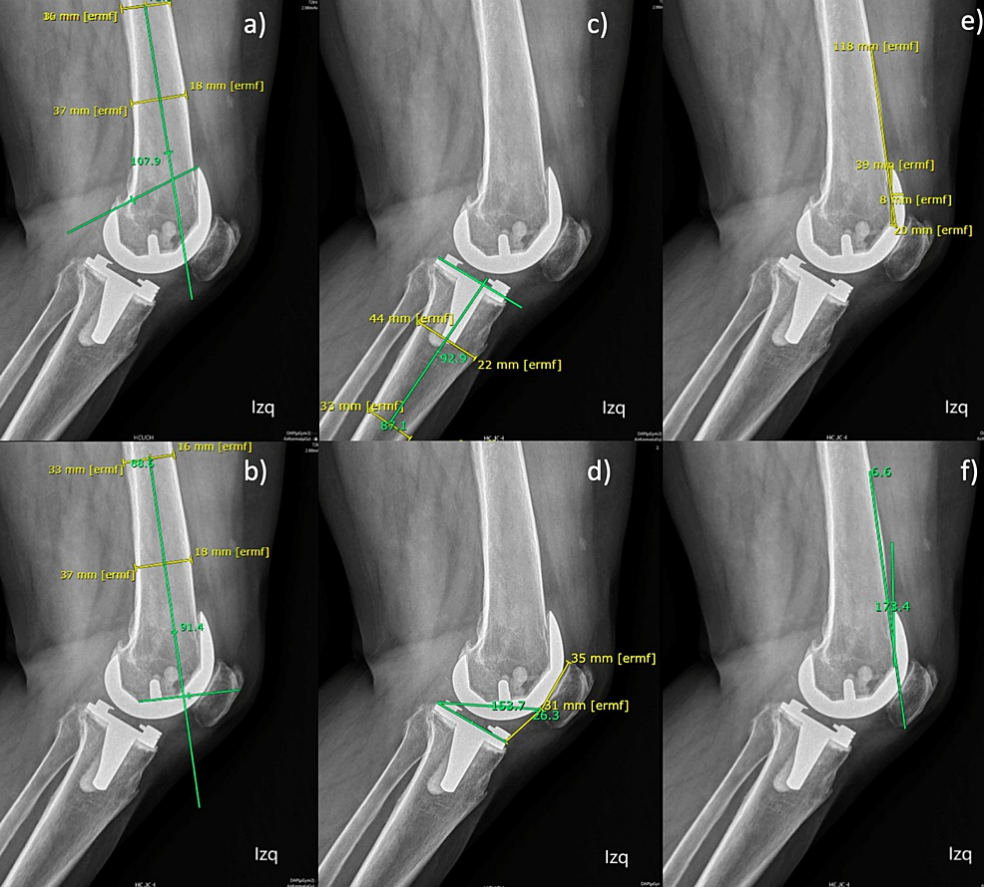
Lateral knee radiograph (a) Sagittal anatomic lateral view femoral component alignment 1; (b) Sagittal anatomic lateral view femoral component alignment 2; (c) Sagittal anatomic lateral view tibial component alignment; (d) Patela Height (yellow line). Patela Height 2 (green line); (e) Offset femoral anterior; (f) The anterior inclination angle of the femoral component
